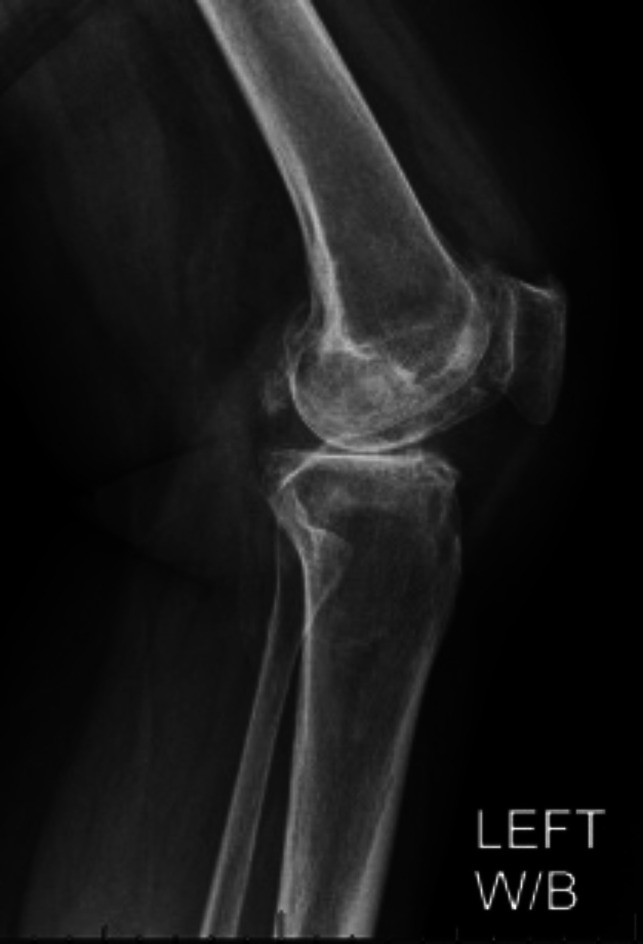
Lateral radiograph of the left knee.
